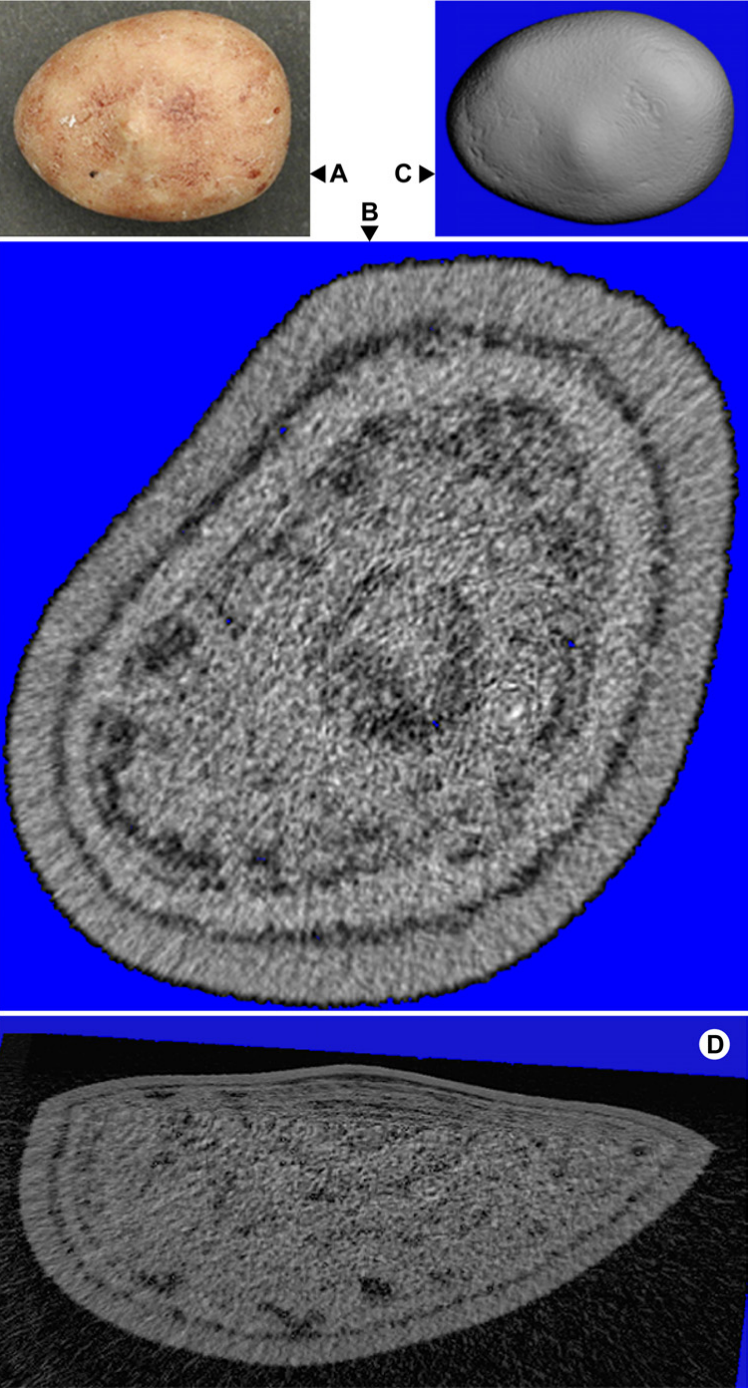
Imaging and software power of micro CT. Pure COM stone viewed by conventional photography (A) and micro CT (B-D). B shows a typical micro CT image slice, C a 3-D reconstruction of the stone surface, and D a wedge cut that displays the internal 3-D morphology of the stone.
